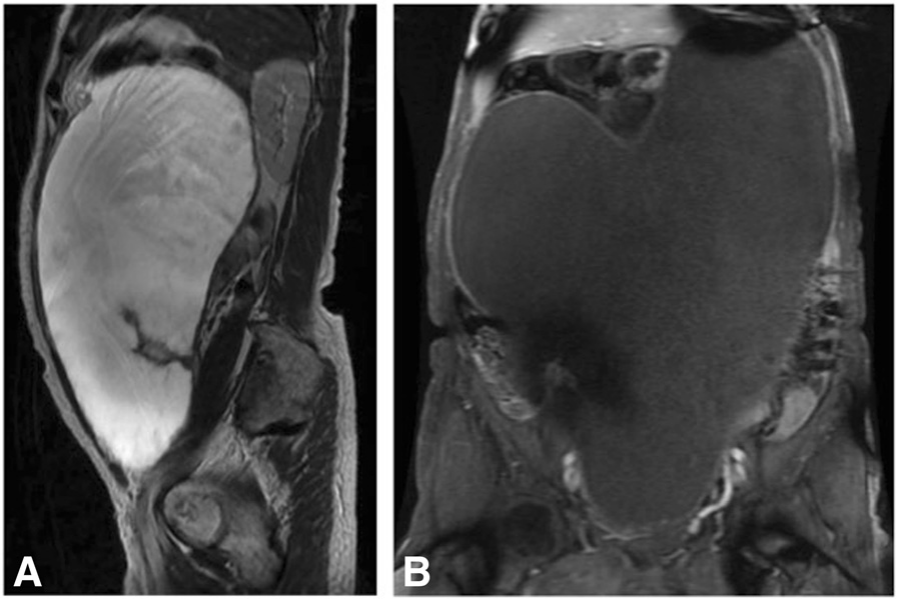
Magnetic resonance imaging demonstrated a giant unilocular cystic mass with high signal intensity on T2-weighted images (a) and intermediate signal intensity on T1-weighted images (b)
